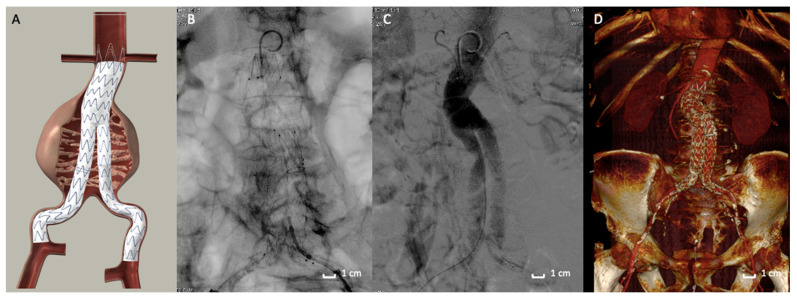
Traditional stent-graft design. (A) Picture of the endograft deployed in Abdominal Aortic Aneurysm. Traditional Endovascular aneurysm repair (EVAR) devices employ self-expanding stent structures to promote graft-to-aortic wall apposition (seal) and fixation. (B) Intraoperative fluoroscopic image of the endograft deployed in the abdominal aortic aneurysm (AAA). (C) Angiographic acquisition that reveals a good exclusion of the AAA and the good patency of the endograft. (D) 3D volume rendering of Computed Tomography Angiography at 1-month follow-up that shows the correct aneurysm exclusion.
